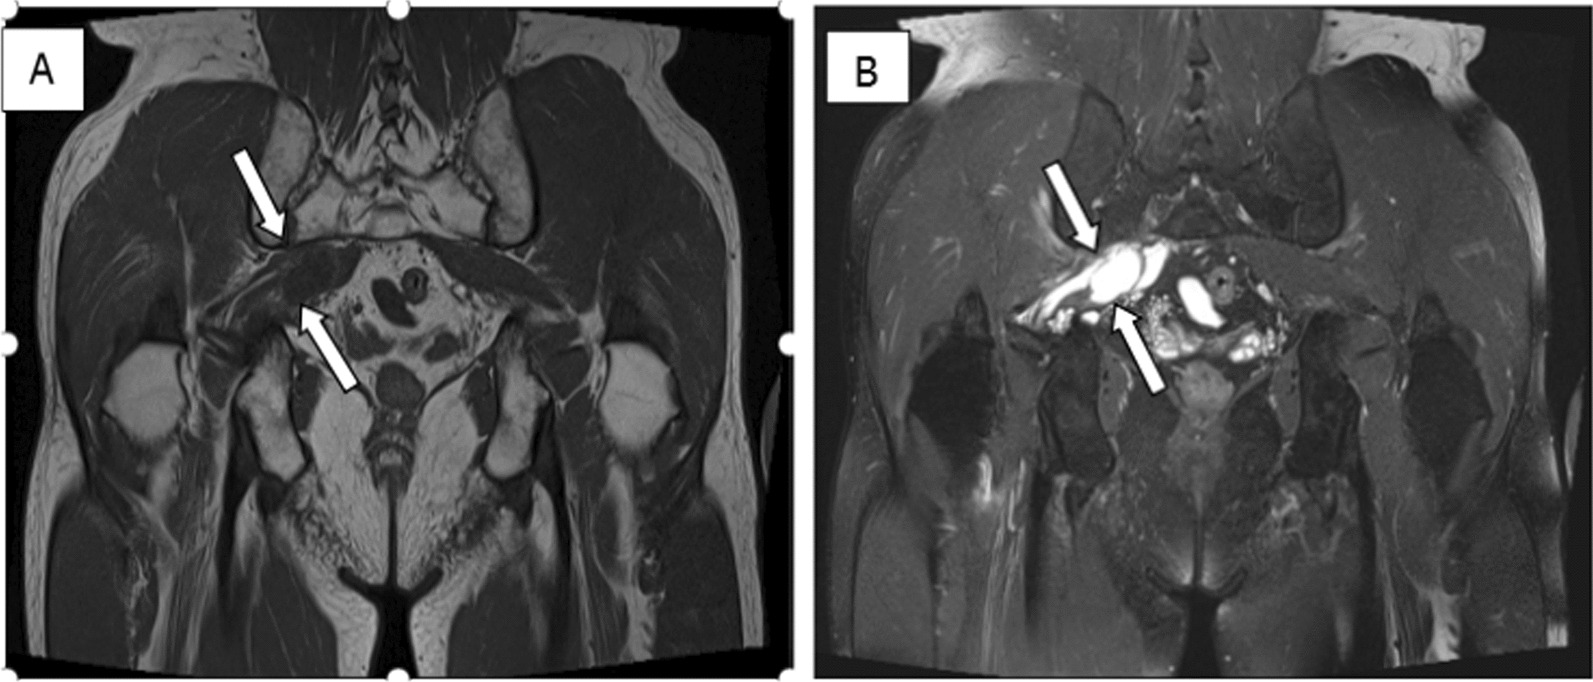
MRI demonstrating multiple cysts (arrows) in piriformis muscle region with long T1 (A) and long T2 (B) signals
